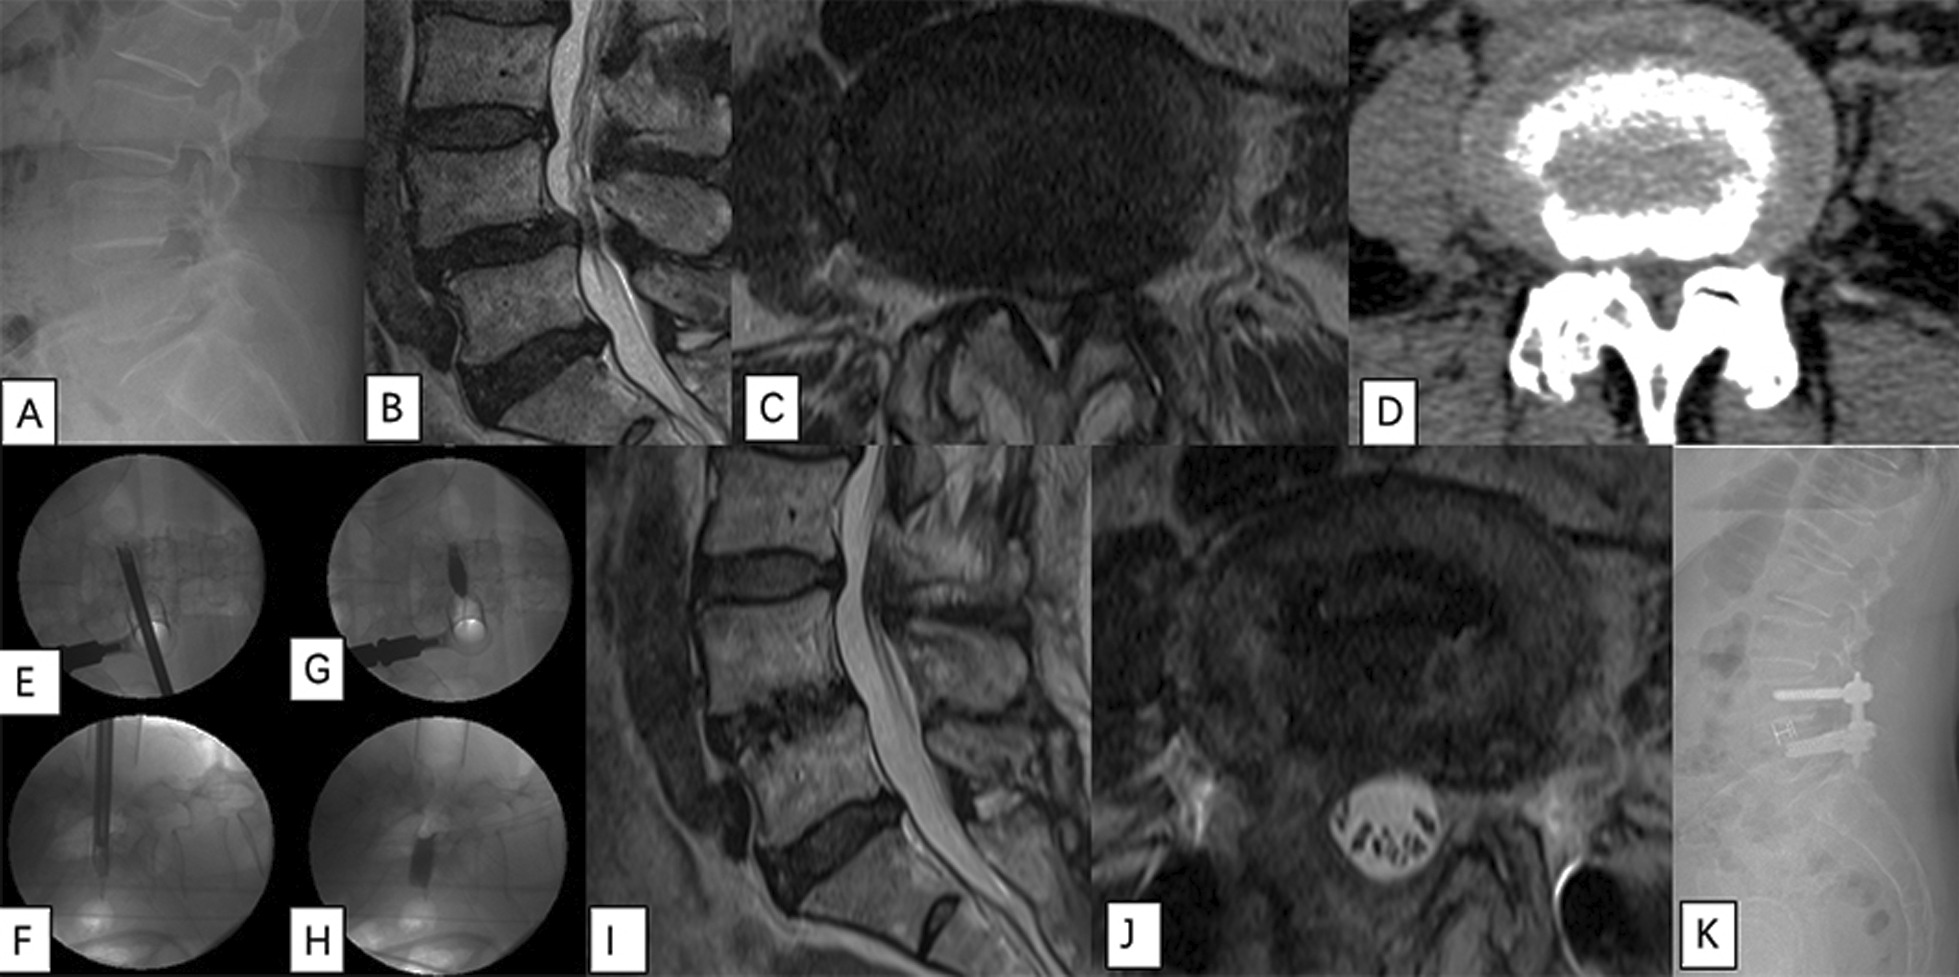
An age 67 female patient underwent endoscope-assisted minimally invasive transforaminal lumbar interbody fusion with fluoroscopy-guided technique at L4–5 level. A X-ray images show lumbar spondylolisthesis at L4–5. B, C MR images show severe central stenosis at L4–5. D CT image show severe stenosis at L4-5 and bilateral facet joint degeneration. E, F Disc space preparation using endoscope. G, H C-arm images were taken in anteroposterior and lateral views to confirm extent of discectomy. I, J 1-year postoperative MR images. K 1-year postoperative X-ray image
